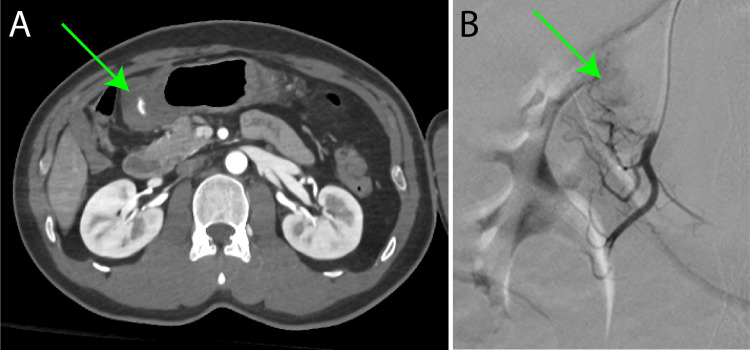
Imaging at the time of injury due to multiple traumas. (A) Contrast-enhanced computed tomography (CT) revealed active bleeding from the duodenum (green arrow). (B) Angiography confirmed the same finding (green arrow). Hemostasis was subsequently achieved using an embolic material.
